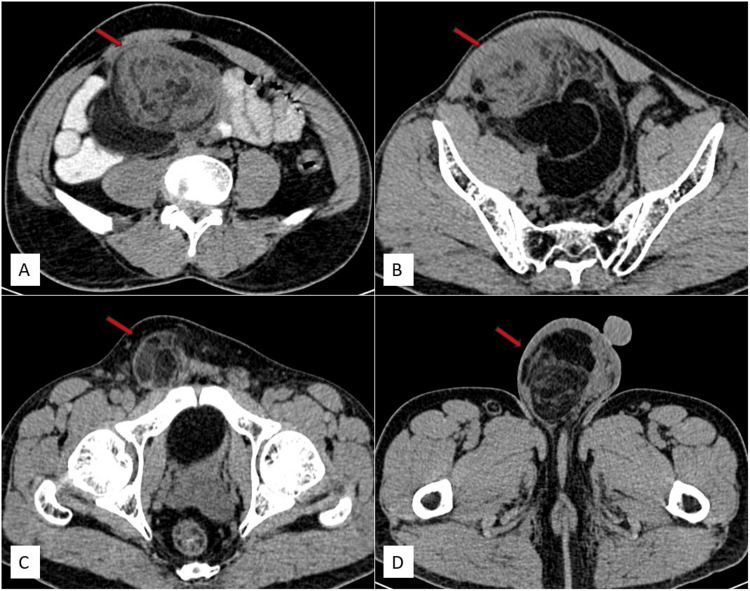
Axial non-contrast CT images depict a solid mass of heterogeneous density, showing both lipomatous and non-lipomatous components, in the retroperitoneum. It extends inferiorly into the pelvis, right inguinal region, and right scrotal sac up to the upper pole of the right testis. Axial non-contrast CT images reveal a mass of heterogeneous density in the retroperitoneum (red arrow) (A), extending inferiorly into the pelvis (red arrow) (B) and right inguinal region (red arrow) (C) and reaching the right scrotal sac up to the upper pole of the right testis (red arrow) (D). CT: computed tomography
